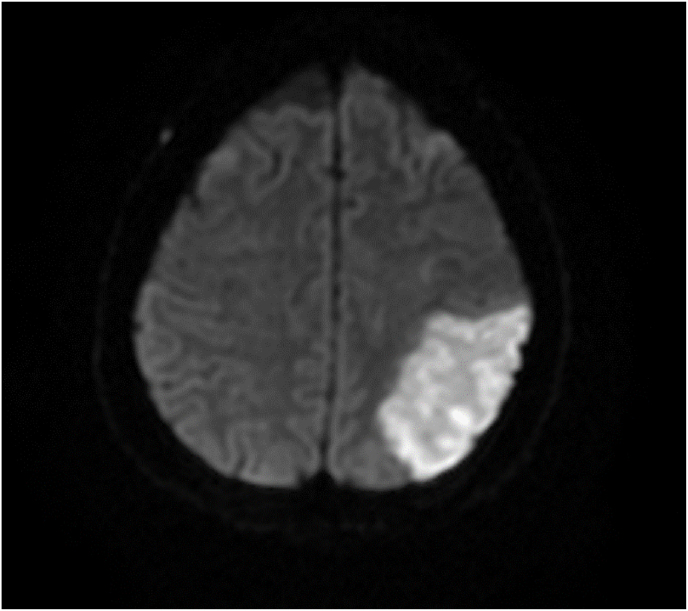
MRI-cerebrum:Axial diffusion weighted image showing acute left middle cerebral artery infarct.
